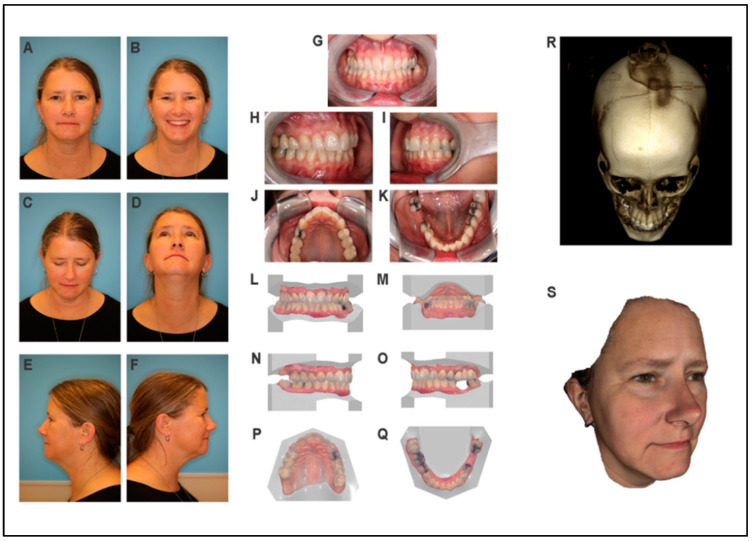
Clinical assessment of the unaffected mother of the proband. (A–F) Two-dimensional photographs of the proband’s mother. (G–K) Intraoral photographs. (L–Q) Intraoral scan images. (R) Three-dimensional CBCT scan image. (S) Three-dimensional soft tissue image.
